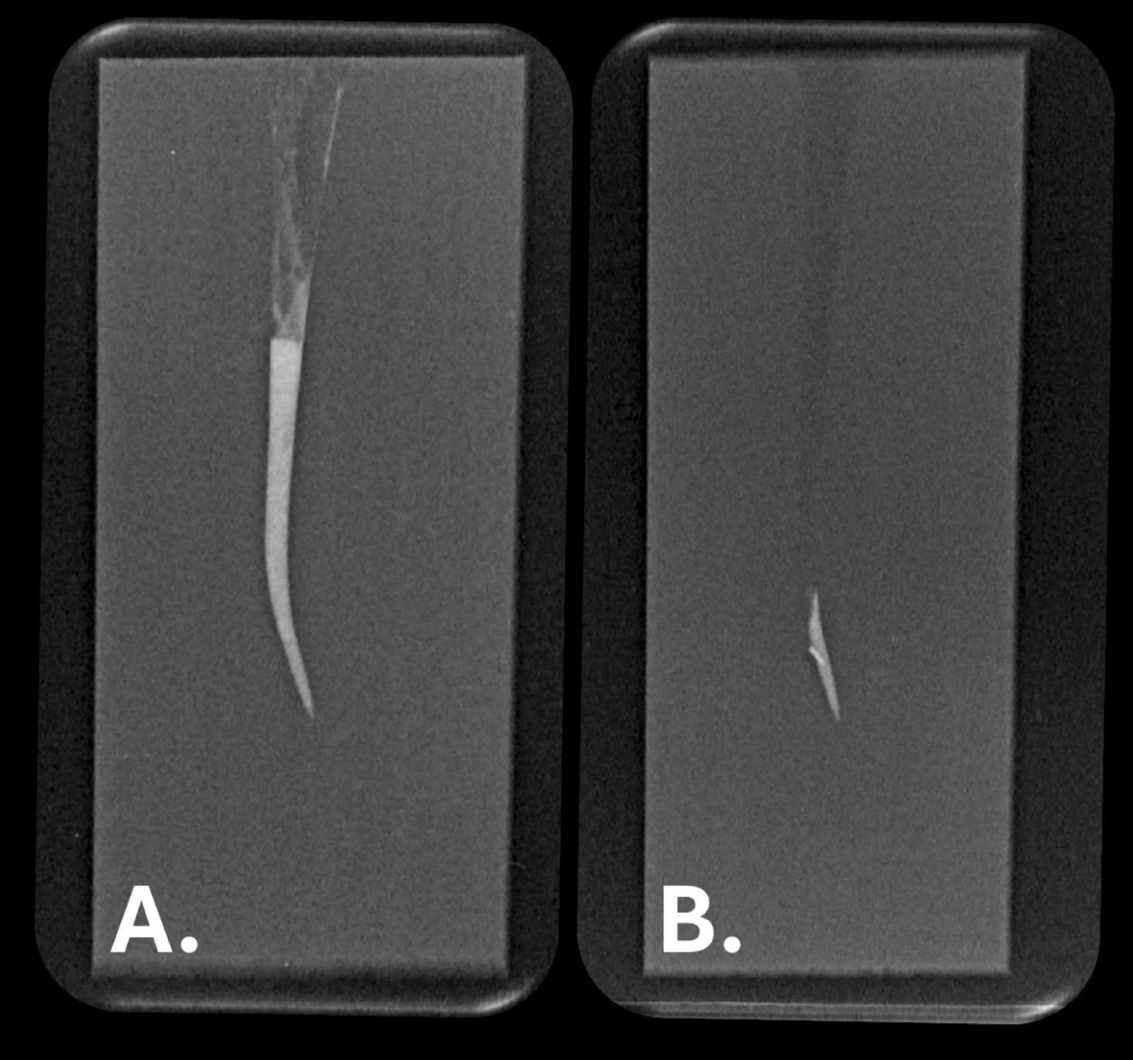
Representative radiographs of plastic blocks: (A) Following filling of the canal with MTA and (B) after attempted removal of the MTA root canal filling.
